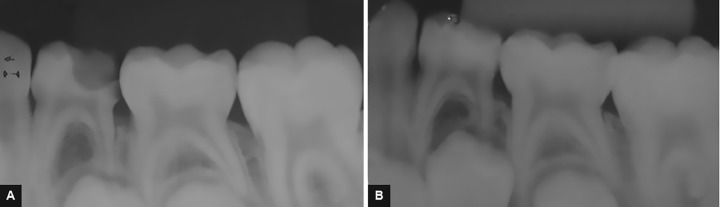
PCR on tooth #74. (A) Initial X-ray; (B) postoperative radiograph in the follow-up period
